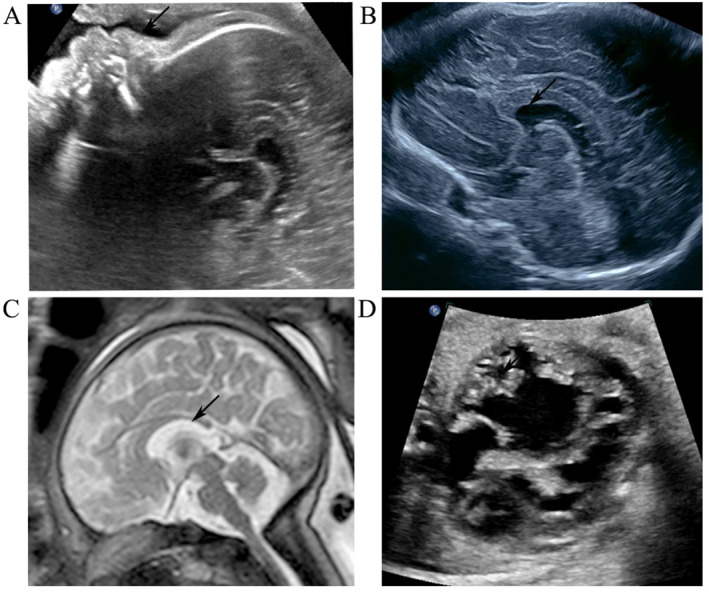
(A) Arrow indicates the hypoplastic nasal bone detected by the ultrasound. (B) The arrow indicates the dilated anterior horns of the lateral cerebral ventricles detected at the routine fetal morphology scan at 23 GW. (C) The arrow points to a short corpus callosum identified by fetal MRI. (D) Fetal ultrasound at 35 GW showed severe cardiomegaly, with globularly dilated left ventricle (LV). The arrow indicates prominent trabeculae.
